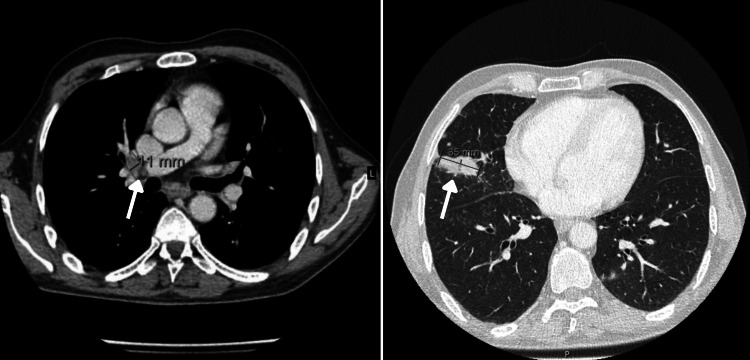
Thoraco-abdominal CT scan revealing pulmonary lymphadenopathy (left) and a lung cancer lesion (right)
